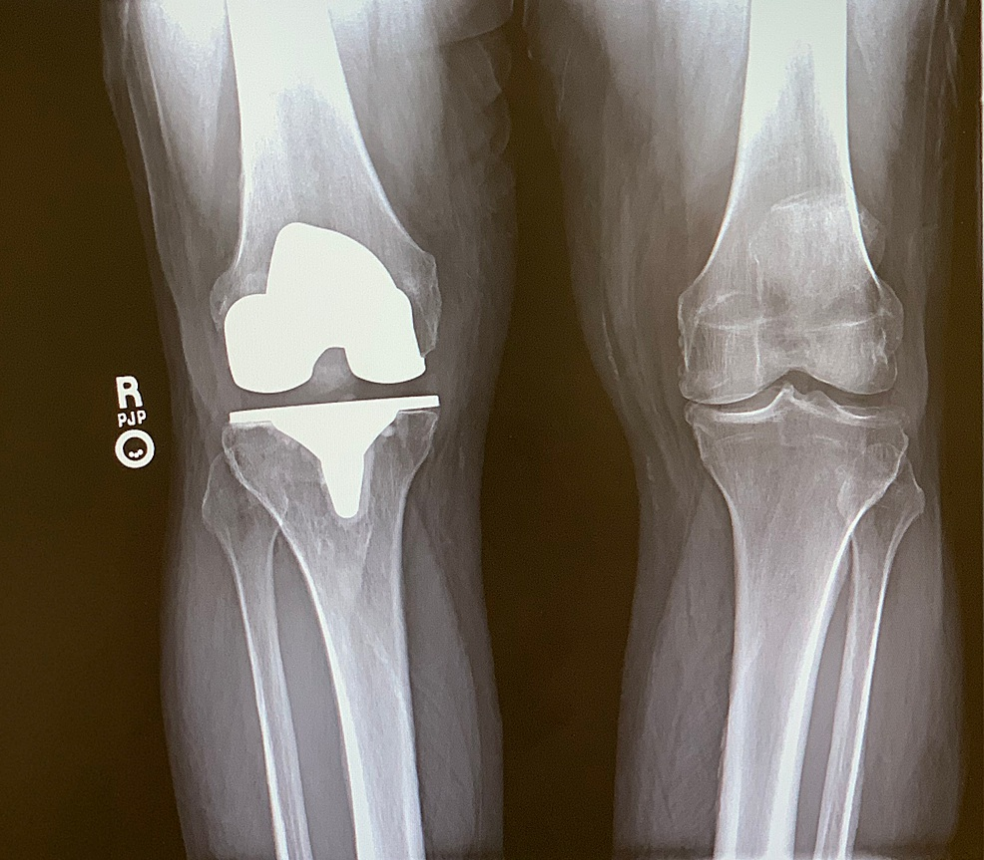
Preoperative standing AP radiographs of the bilateral knees showing lack of cement adherence along the medial aspect of the tibial baseplate with early subchondral osteolysis. AP: anteroposterior
